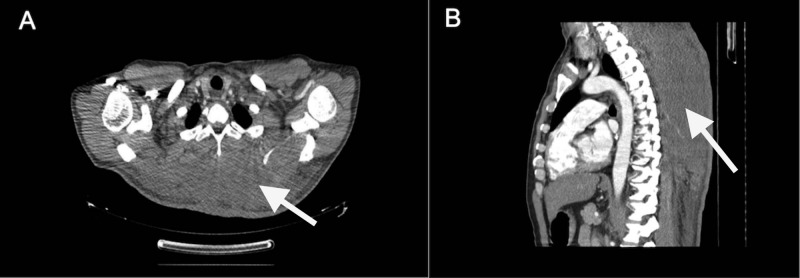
Chest CT Scan A chest CT scan with contrast.  White arrows showed an undefined heterogeneous mass dependent on the soft tissues in the posterior thorax wall, with infiltration signs in the muscular plane and the density alteration of the subcutaneous cellular tissue and skin. A) Axial view. B) Sagittal view.
